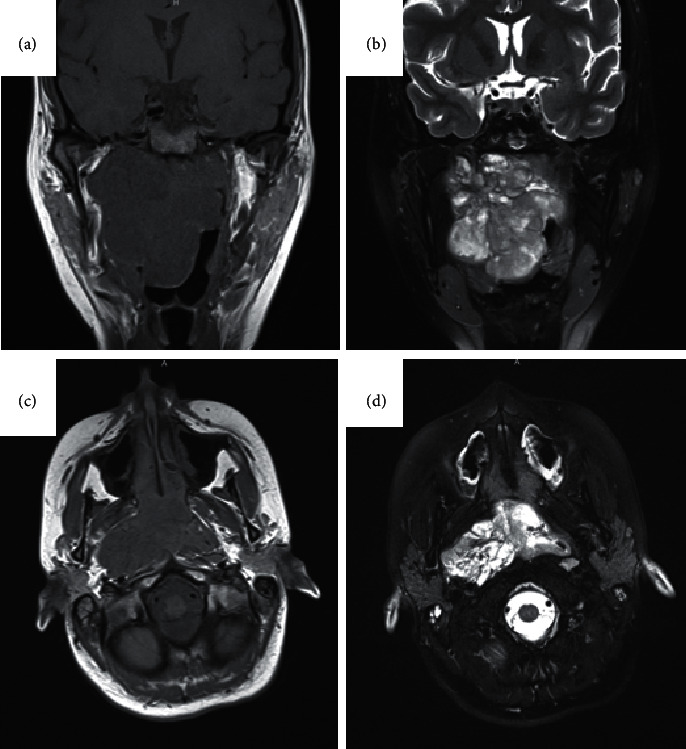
MRI findings of nasopharyngeal mass. (a) T1-weighted MRI in the coronal plane (b) T2-weighted coronal plane (c) T1-weighted axial plane (d) T2-weighted axial plane.
